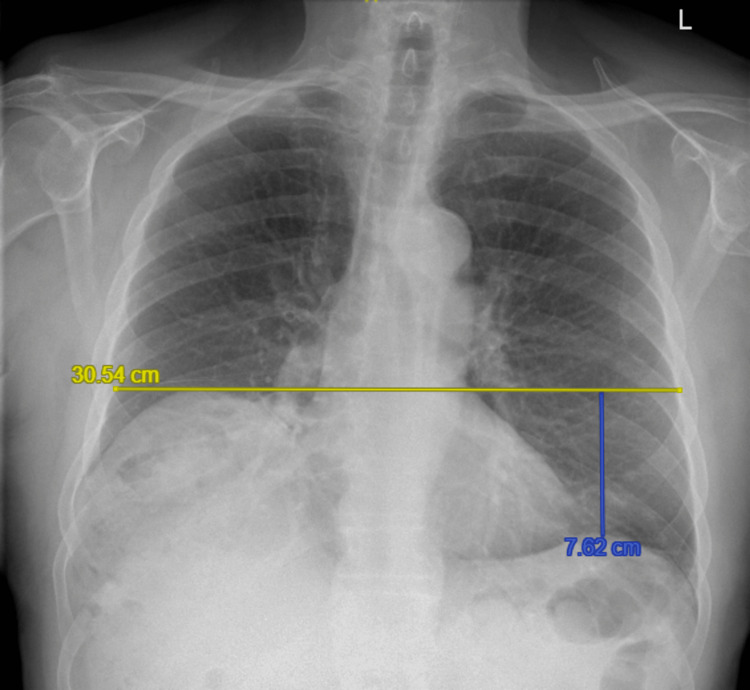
Chest X-ray on presentation in 2025, showing the level of elevated right hemidiaphragm and distance from left hemidiaphragm. Yellow line indicates the higher level of the right hemidiaphragm while the blue line is the height of the right hemidiaphragm in relation to the left hemidiaphragm.
